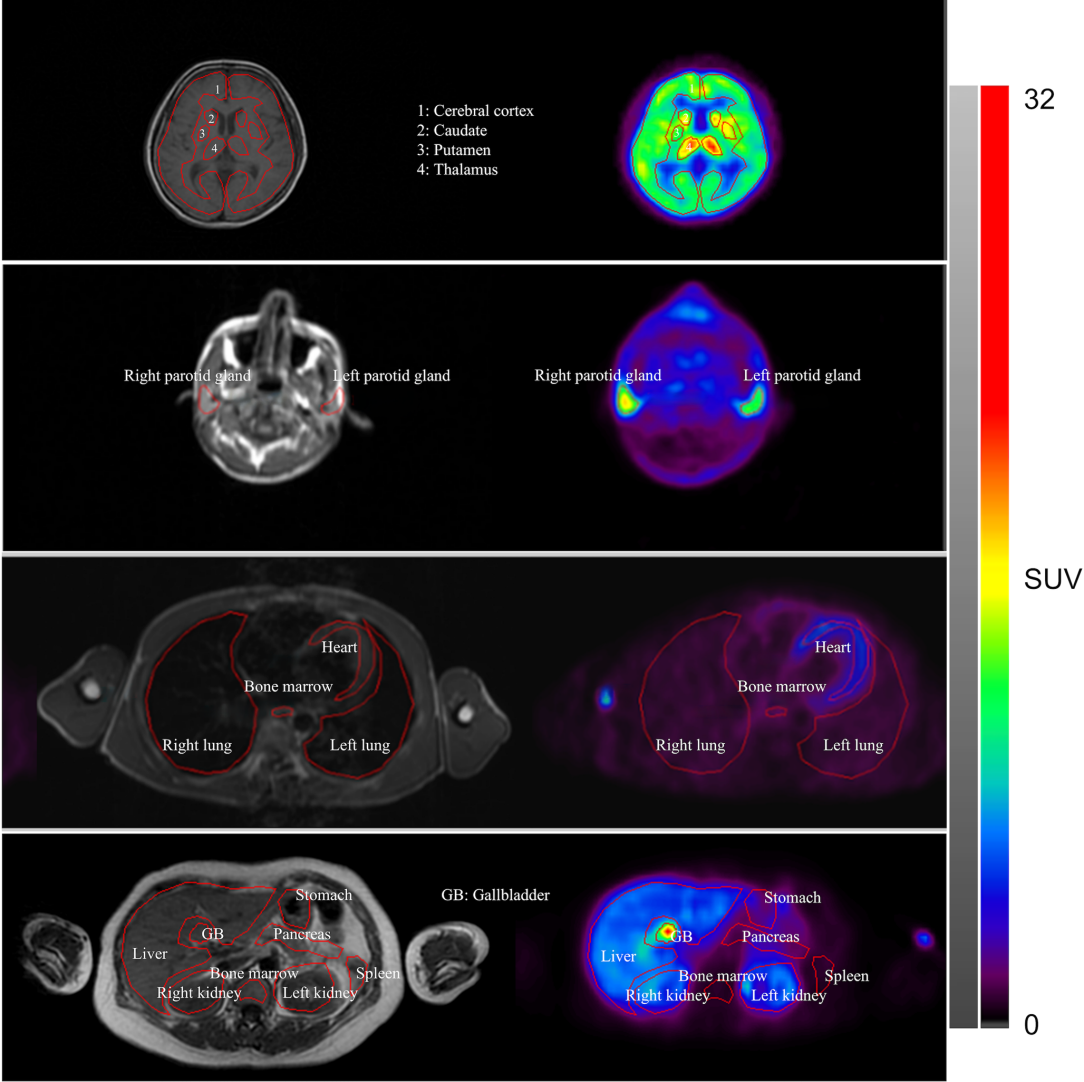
Representative [^18^F]SMBT-1 PET and MR images with examples of region of interest (ROI). In the axial plane, MRI (left) and PET images (right) depict the delineation of interest (ROIs) at the head (brain and parotid glands), chest, and abdominal levels. These ROIs were manually outlined on the PET images, with visual guidance provided by the concurrent display of MRI images on dual monitors, facilitating a side-by-side comparison of both imaging modalities. Abbreviations: MRI, magnetic resonance imaging; ROIs, regions of interest; PET, positron emission tomography
